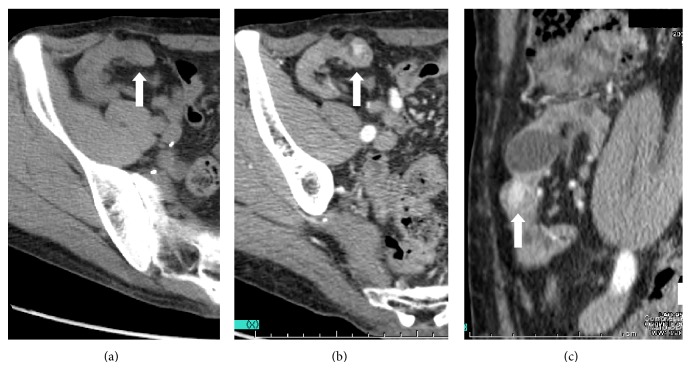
Computed tomography images. (a) A noncontrast computed tomography (CT) scan in the horizontal view, revealing no tumor in the kidney graft. (b) and (c) Intravenous contrast-enhanced CT scans in horizontal (b) and sagittal (c) views showing an enhanced solid tumor in the kidney graft (*white arrows*).
